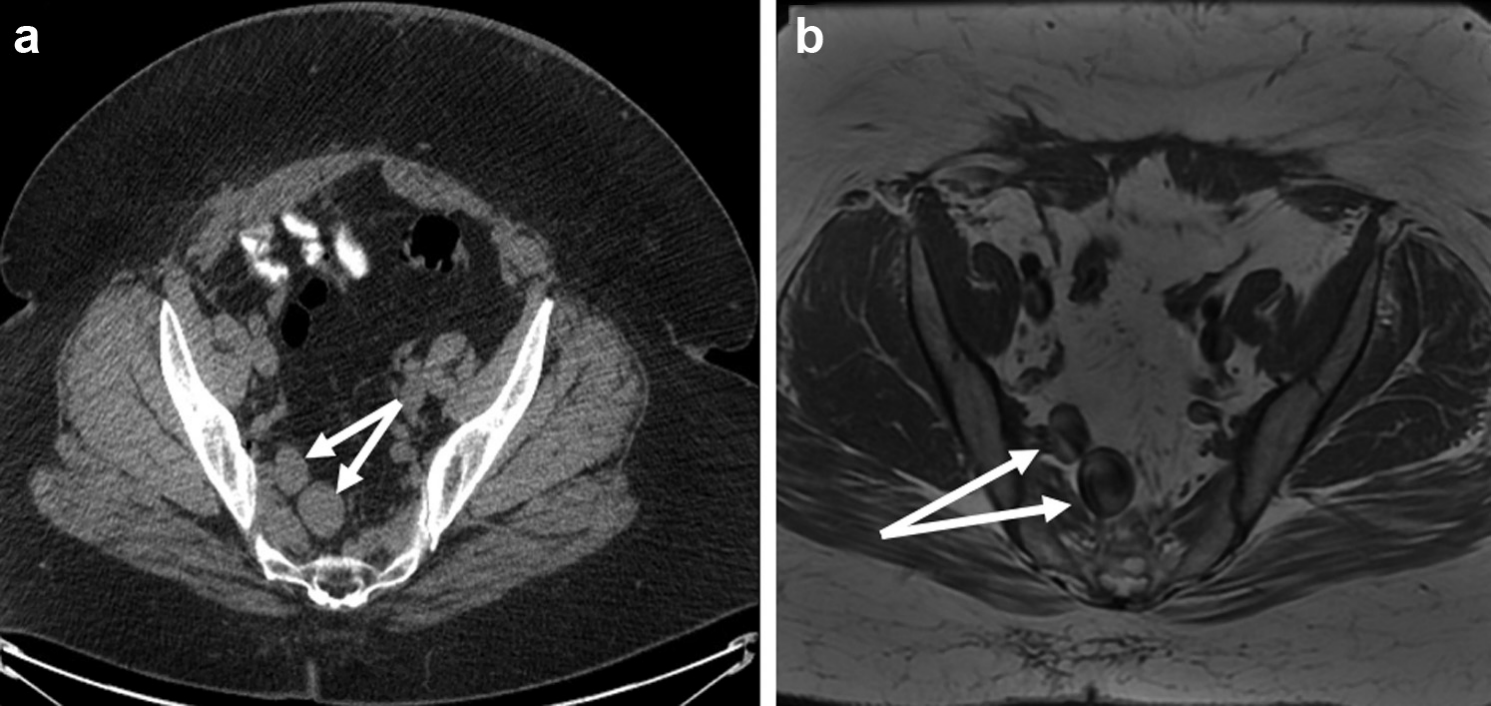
Computed tomography scan (A) and magnetic resonance imaging (B) demonstrating two right sided iliac vein aneurysms measuring approximately 2.5 and 3.0 cm with varicosities (arrows).
